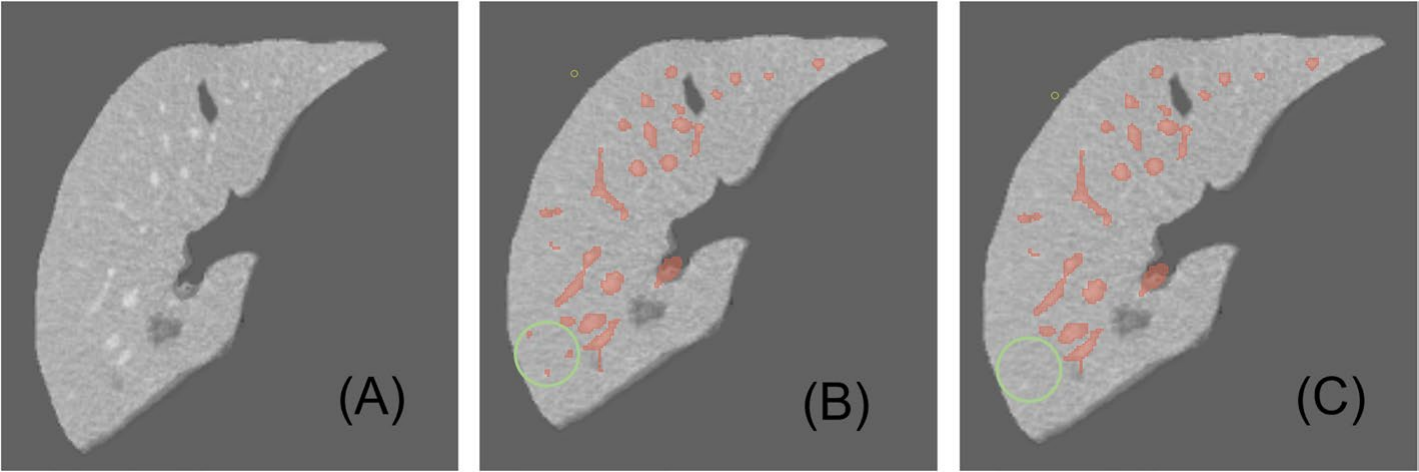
Effect of our pre-processing, (A) is the original, (B) represents before preprocessing, (C) represents the CT after pre-processing
